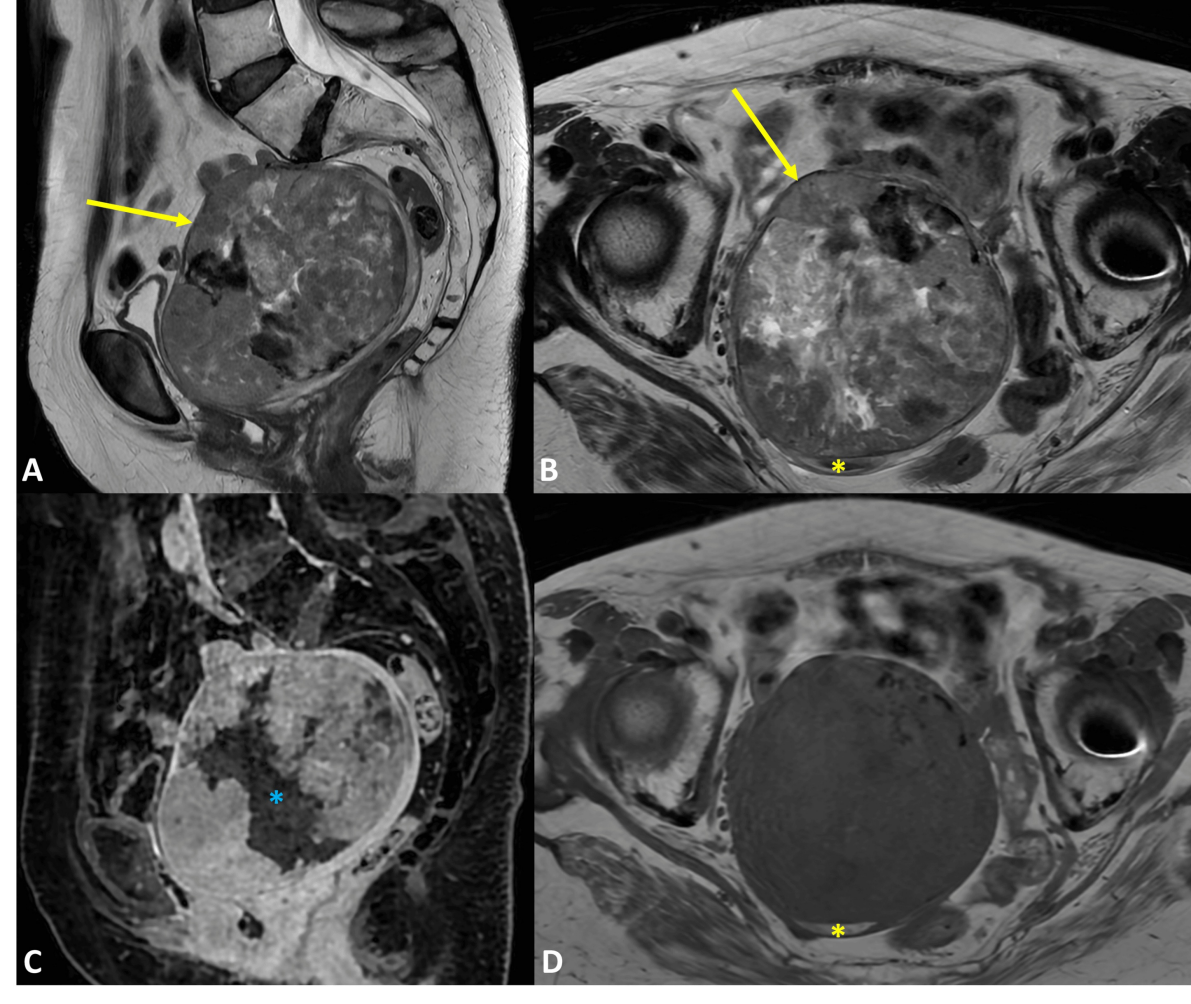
Morphologic MRI sequences of the uterine inflammatory myofibroblastic tumor. Sagittal T2-weighted (A), axial T2-weighted (B), sagittal post-contrast fat-saturated T1-weighted (C), and axial T1-weighted images show a large solid myometrial tumor in the anterior wall of the uterine body (yellow arrow), with intermediate signal and some hypointense areas on T2 images, and regions of chronic hemorrhagic content (yellow asterisk). There is central necrosis (blue asterisk) and no extrauterine extension on the post-contrast fat-saturated T1-weighted image.
